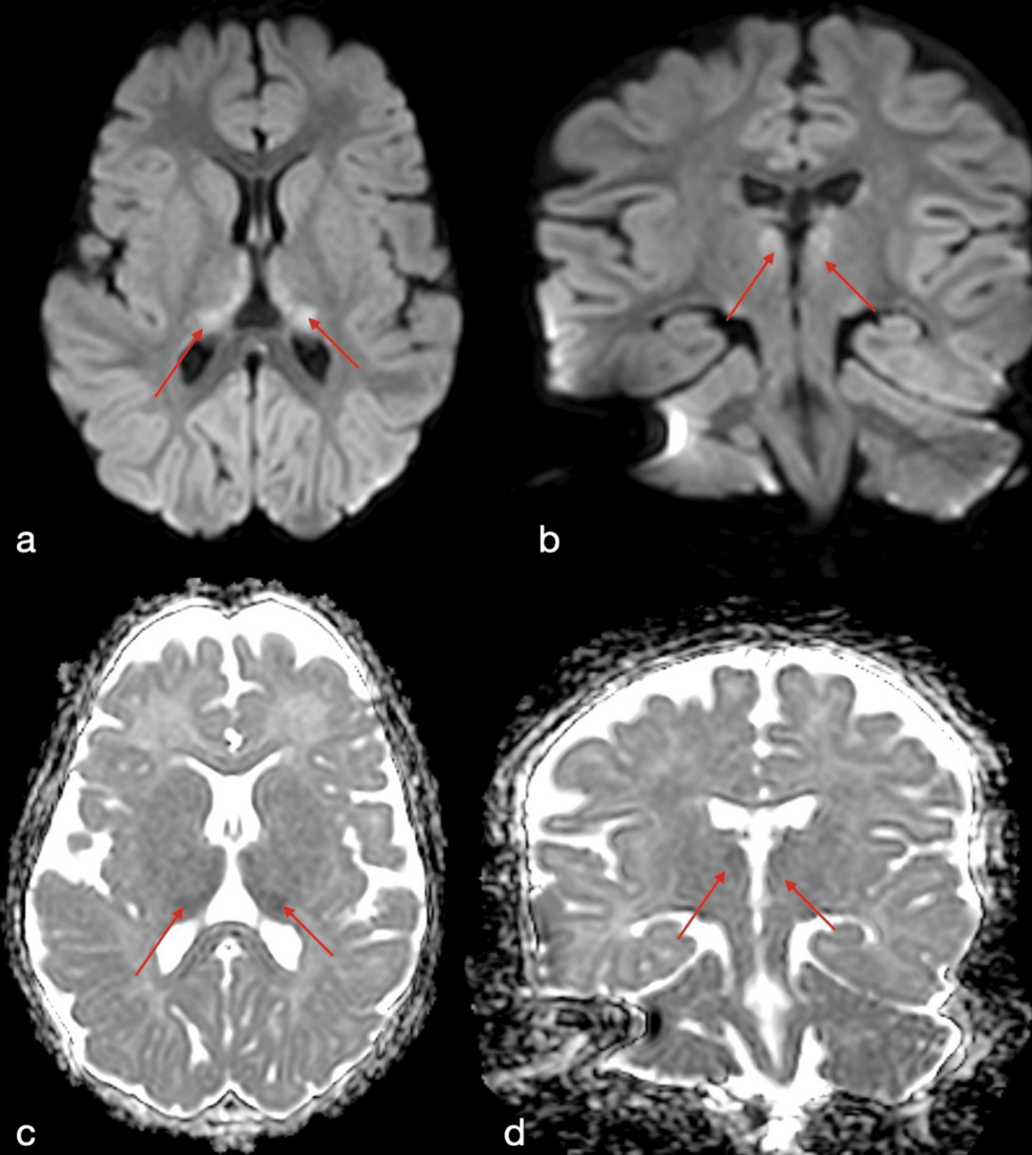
Axial and coronal DWI and ADC brain MRI of the thalami Axial and coronal images demonstrate hyperintense signal on DWI (a and b) and loss of signal on ADC (c and d) in the bilateral paramedian thalami (red arrows) to suggest restricted diffusion. DWI: diffusion-weighted imaging; ADC: apparent diffusion coefficient
